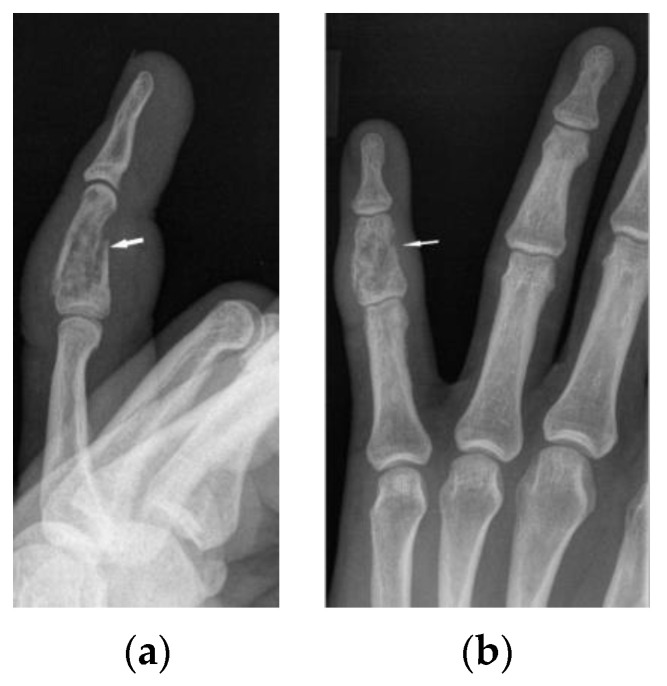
X-ray of the left hand, lateral view (a), PA (b). Osteolytic pattern in the middle phalanx with pathological fracture (white arrows). Soft tissue swelling.
